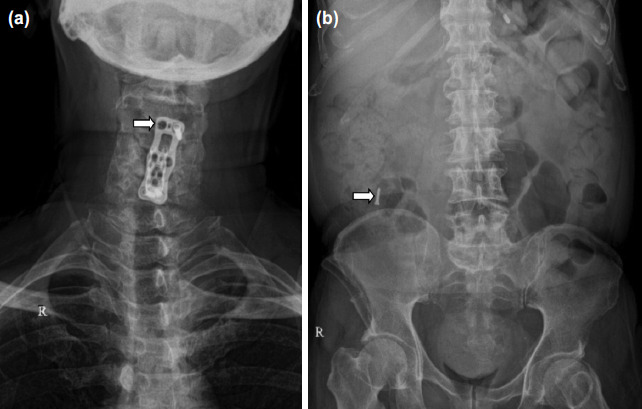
(a) Showing AP radiograph of cervical spine showing missing right sided proximal screw of cervical plate as pointed with white arrow which had earlier backed out, (b) showing AP abdominal radiograph with migrated screw from cervical spine on right side of abdomen as marked with white arrow.
